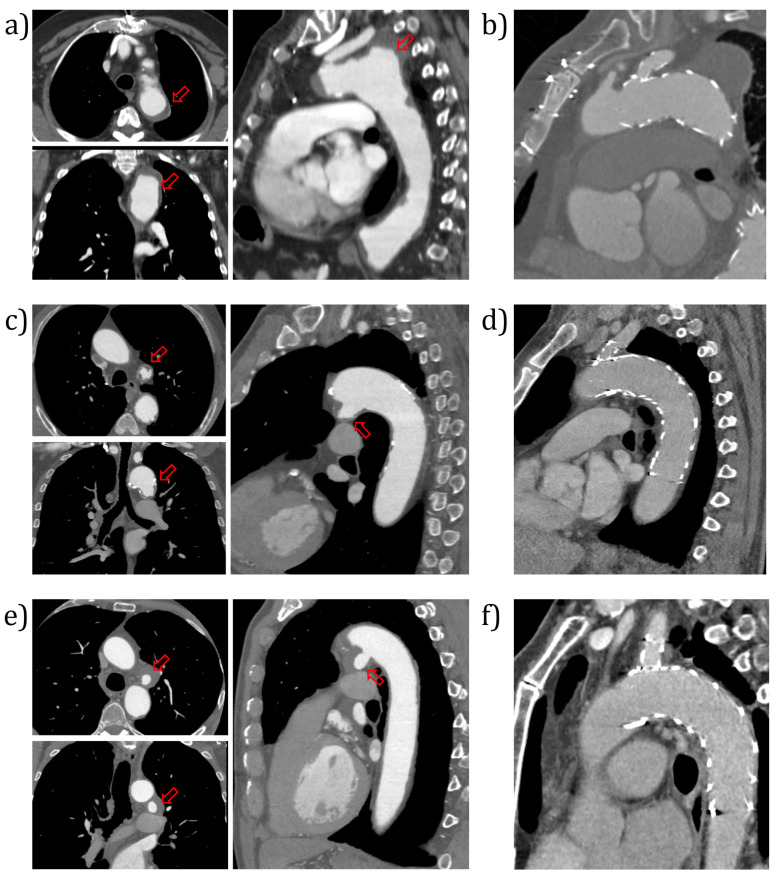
CT acquisitions pre-intervention (a,c,e) and CT acquisitions post-SBSG treatment (b,d,f) for the three cases. PAUs are indicated by red arrows.
